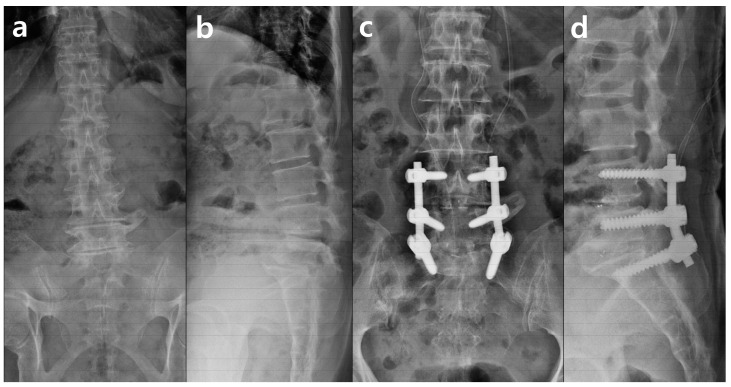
Radiographic image of X-ray at first surgery. (a) Preoperative lumbar AP, (b) preoperative lumbar lateral, (c) postoperative lumbar AP, and (d) postoperative lumbar lateral.
